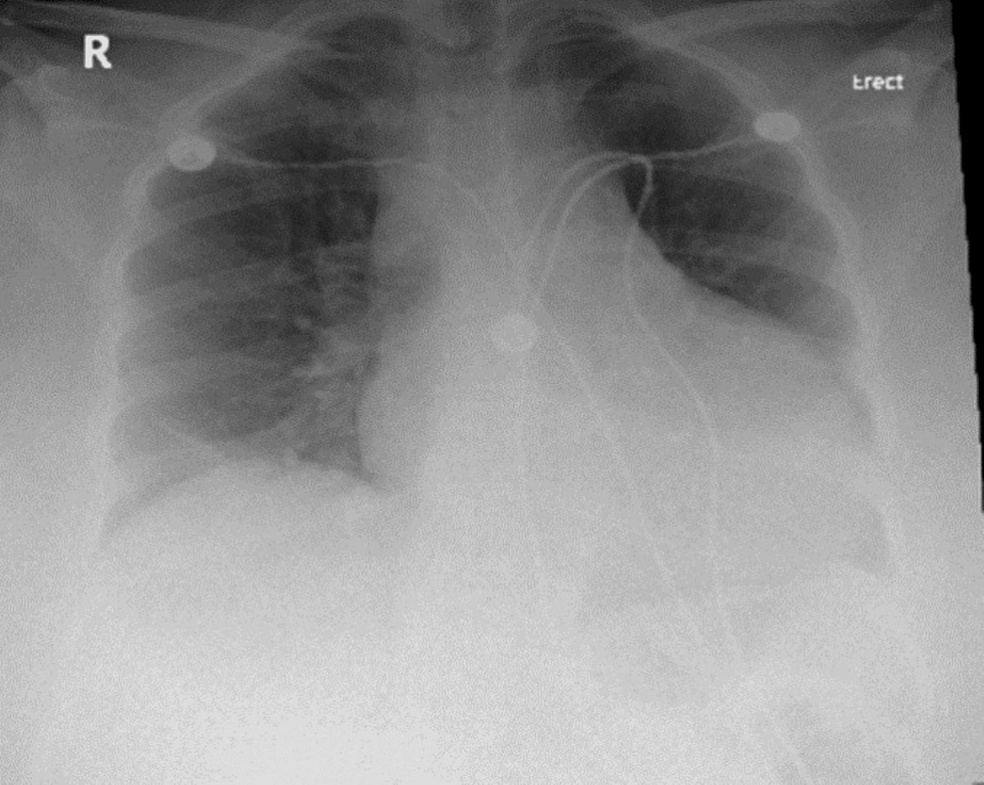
Chest X-ray on day three of admission showing interval cardiomegaly
